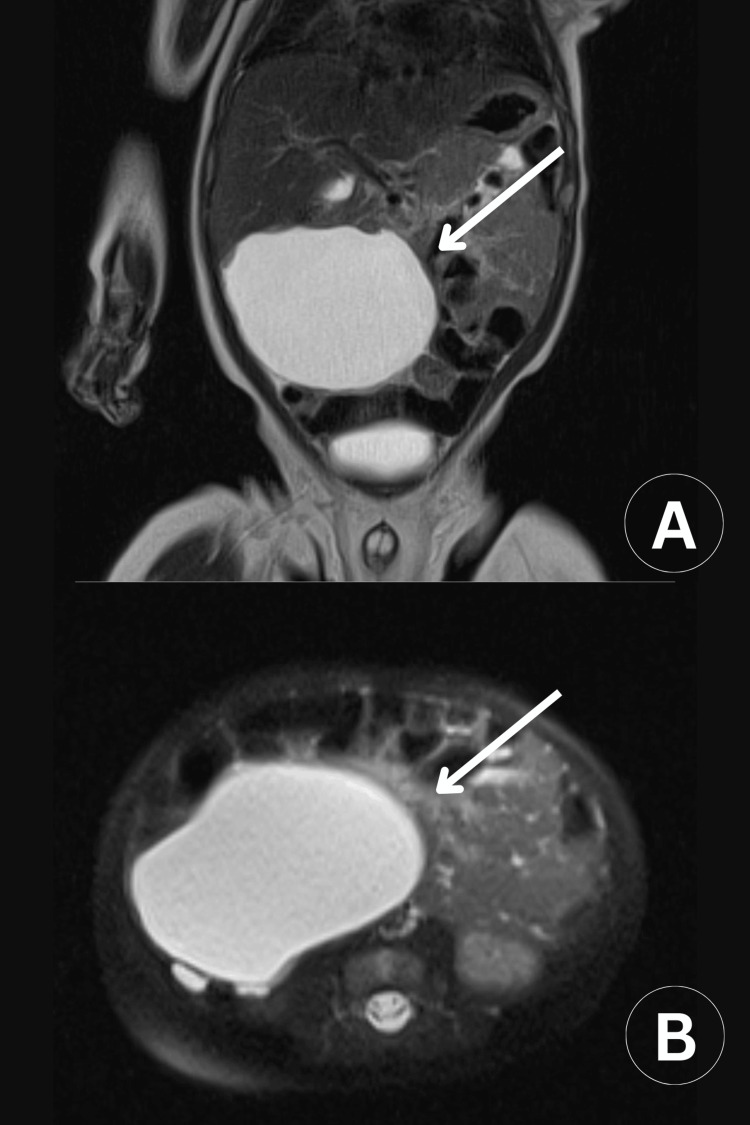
MRI of a large, well-defined cystic lymphangioma. (A) Coronal view with high T2 signal intensity. (B) Axial view with high T2 signal intensity.
